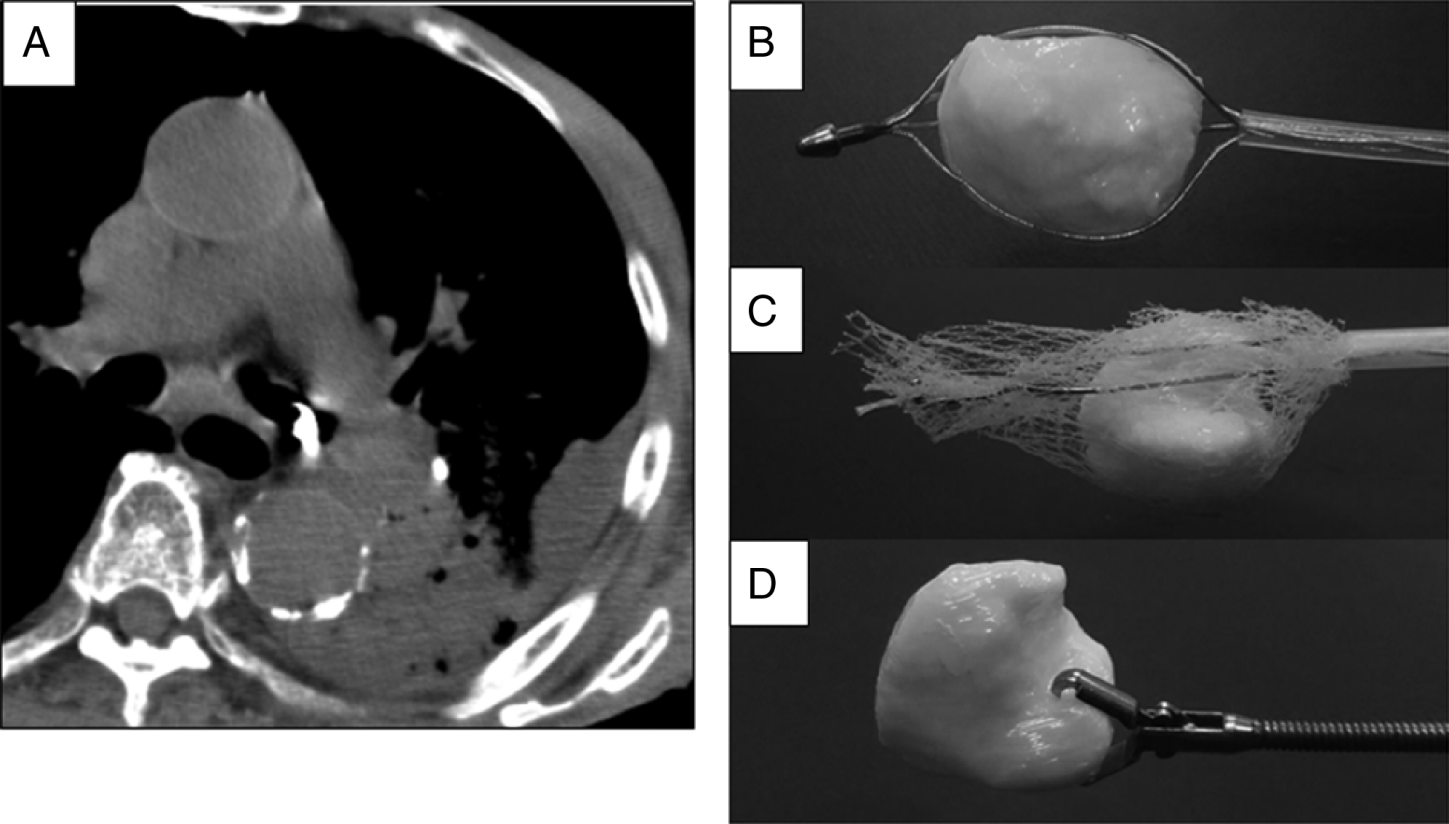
(A) Computed tomography showed a bronchial foreign body in the left main bronchus. We grasped a three‐dimensional model of the foreign body with (B) basket‐type forceps, (C) retrieval net, and (D) shark tooth forceps.
